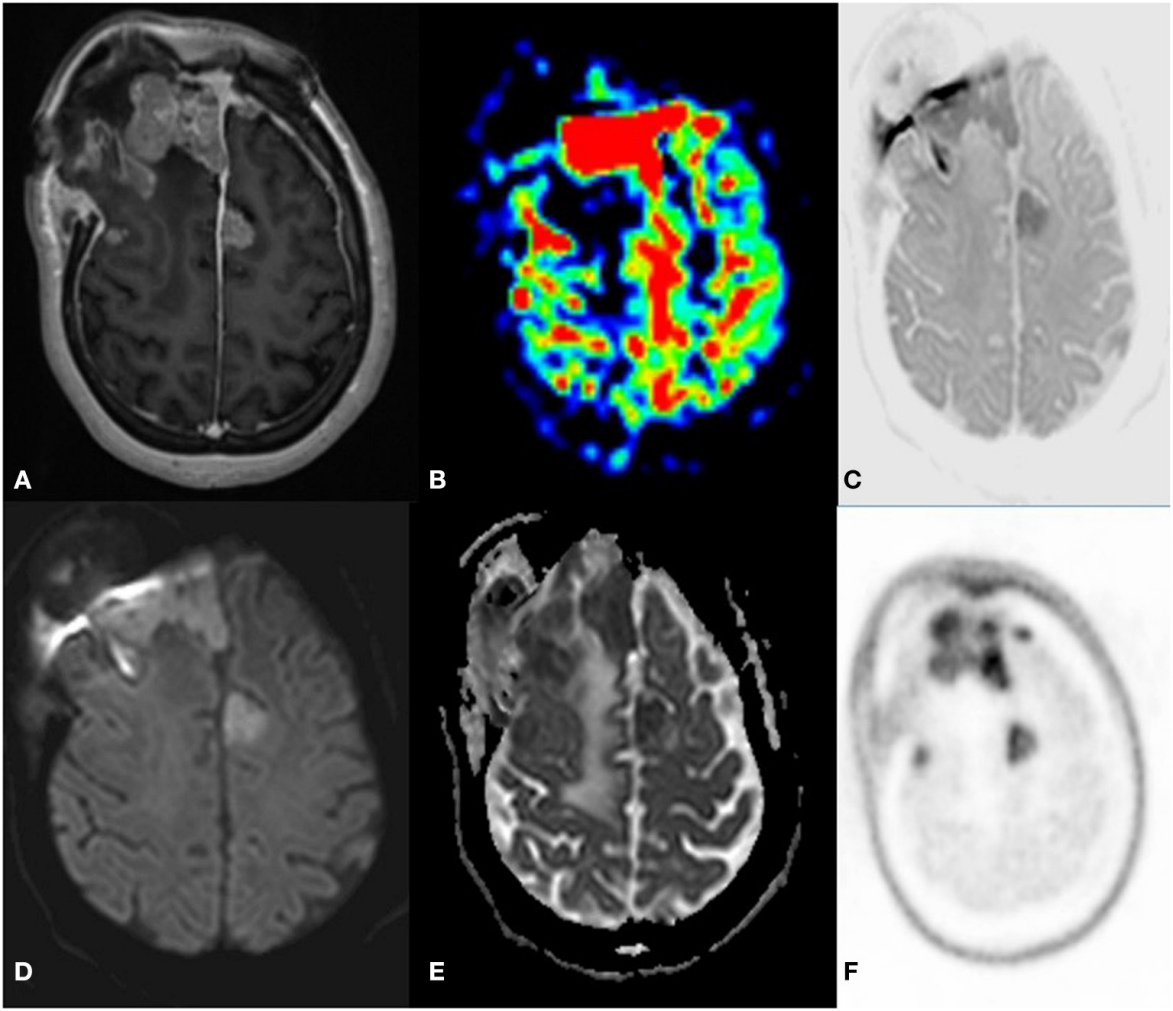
A 33 year old female postoperative, post-radiotherapy case of Embryonal tumor compatible (atypical teratoid rhabdoid tumor) 1 year follow up MRI C-11 Methionine PET axial images post-contrast T1 (A) ASL (B) Inverted DWI (C) DWI b2000 (D) ADC (E) NAC PET (F) showed Multiple enhancing dural based lesions are seen in bilateral frontal convexity and anterior falx with elevated perfusion. The lesion shows DWI restriction with hypermetabolism on PET. There is a match on the inverted DWI image and the NAC PET image, which is suggestive of recurrence.
